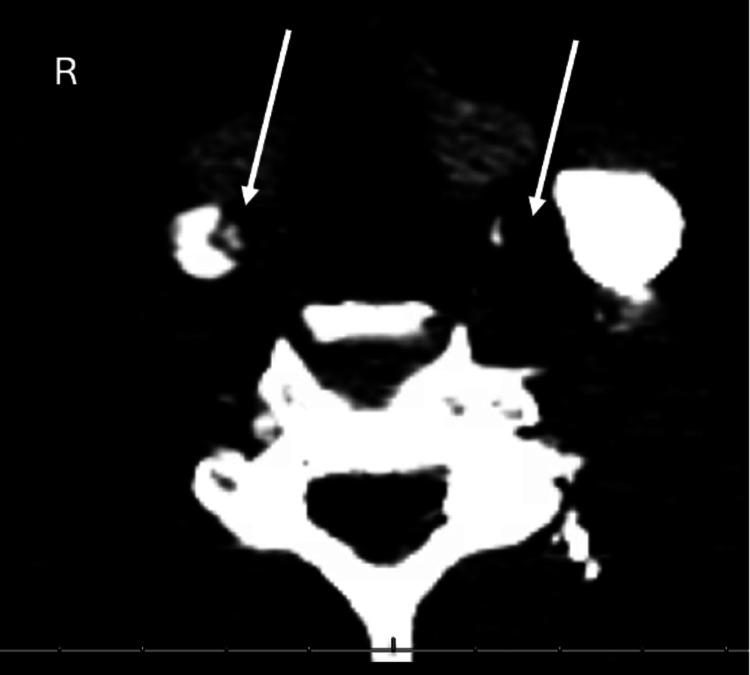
CT angiography of the head and neck revealing the Type A aortic dissection extending into the bilateral common and internal carotid arteries, with near-total occlusion of the left internal carotid artery (white arrows). Image quality is limited due to motion artifact.
